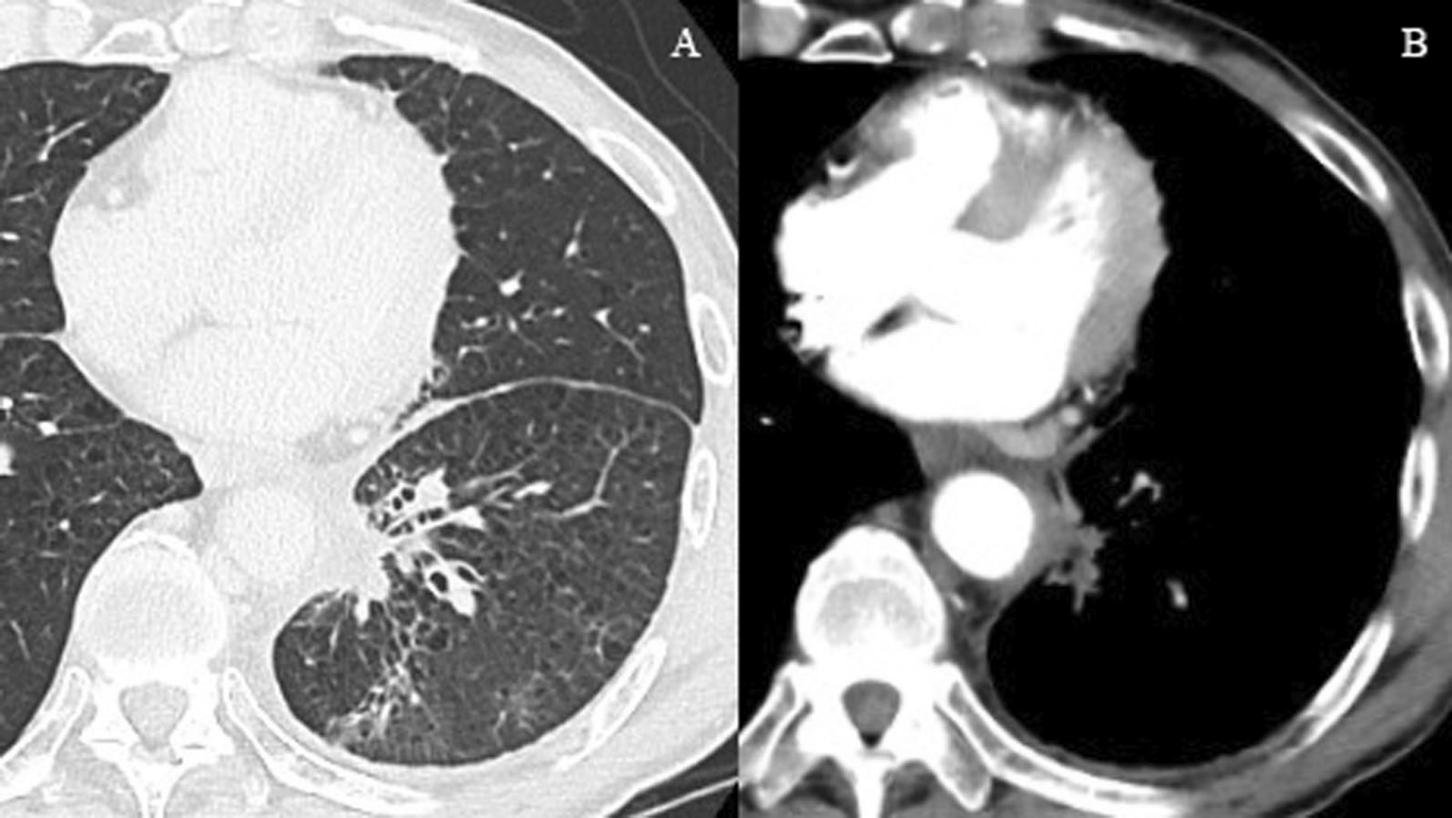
Chest contrast-enhanced CT after neoadjuvant chemoradiotherapy. One month after the chemoradiotherapy, chest contrast enhanced CT showing even reduction of the tumor. CT: computed tomography
